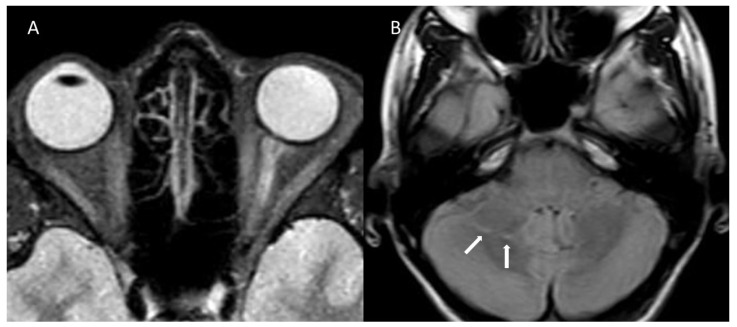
Magnetic resonance imaging scans of case 1. (A) Axial short inversion time recovery sequence demonstrates hyperintensity of the left optic nerve. (B) Axial fluid-attenuated inversion recovery scan of the brain shows two millimetric hyperintense cerebellar foci (arrows).
